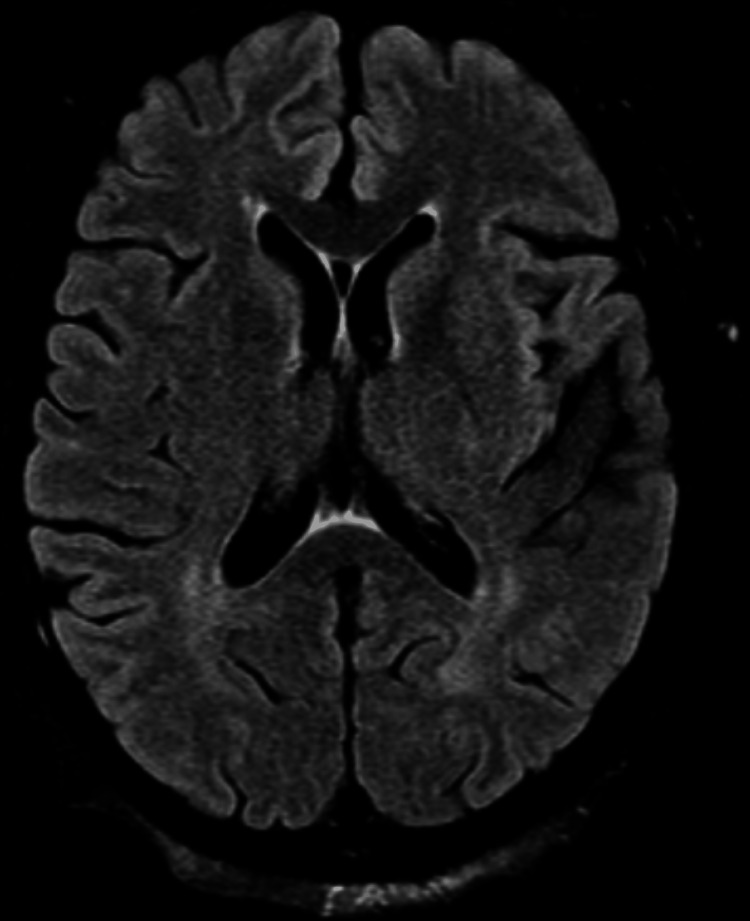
Axial view MRI brain without contrast demonstrating no evidence of acute infarct, midline shift, or hydrocephalus.
